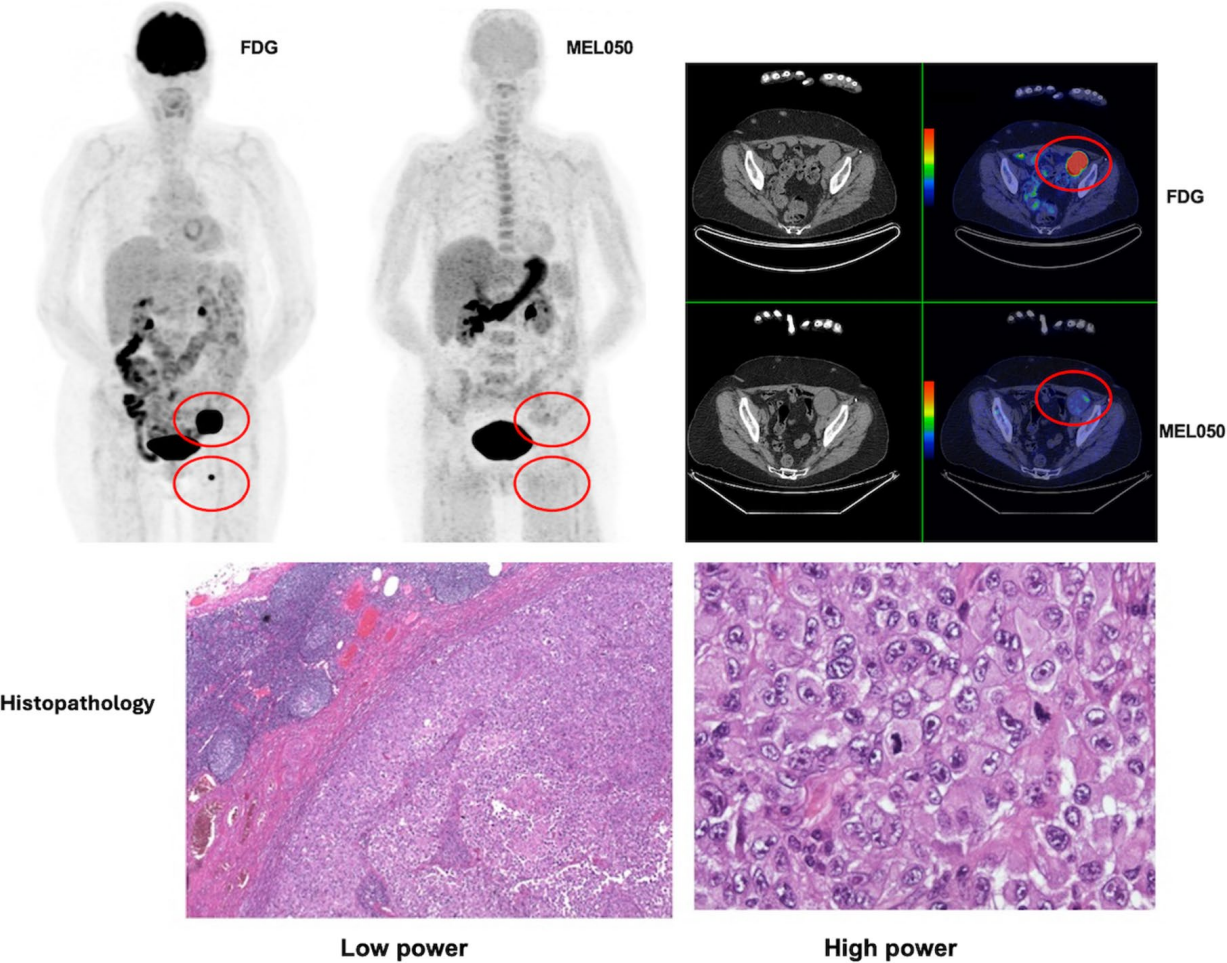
(Patient #1): Left femoral triangle nodal disease subsequently biopsy proven to lack melanin was markedly [18 F]FDG-avid on MIP (far left) and fused transaxial (right upper) but negative on [18 F]MEL050 MIP (centre) and fused PET/CT (right lower). A larger left external iliac node had very patchy [18 F]MEL050 uptake but was primarily negative also
